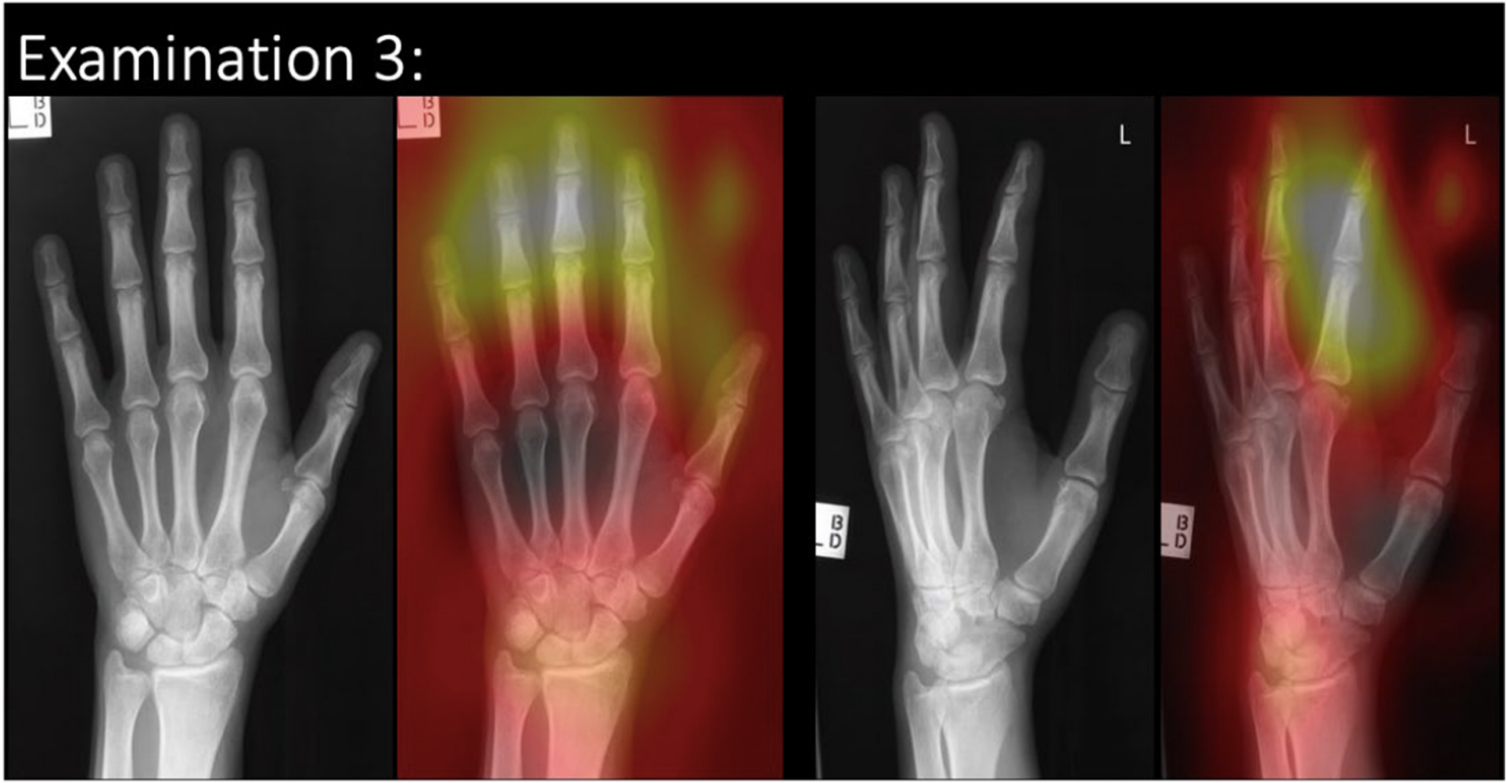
Example of the radiographic image examination shown to the participants.
